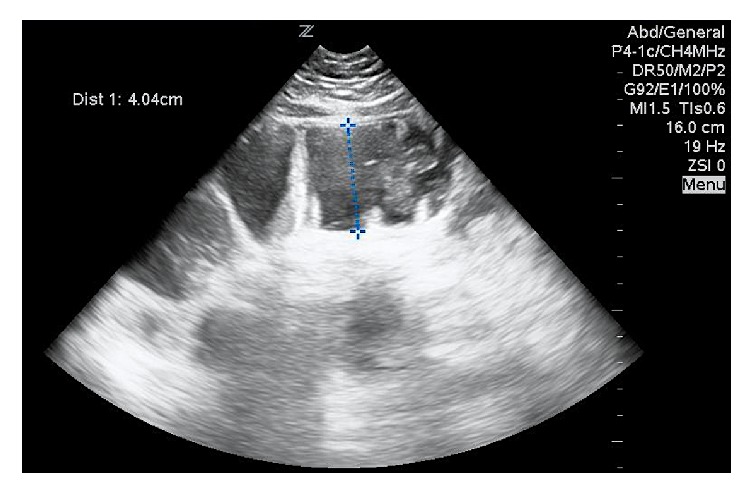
Ultrasound image using a phased array transducer shows a dilated fluid-filled loop of bowel, with a width of more than 4 cm in the left lower quadrant compatible with a small bowel obstruction.
